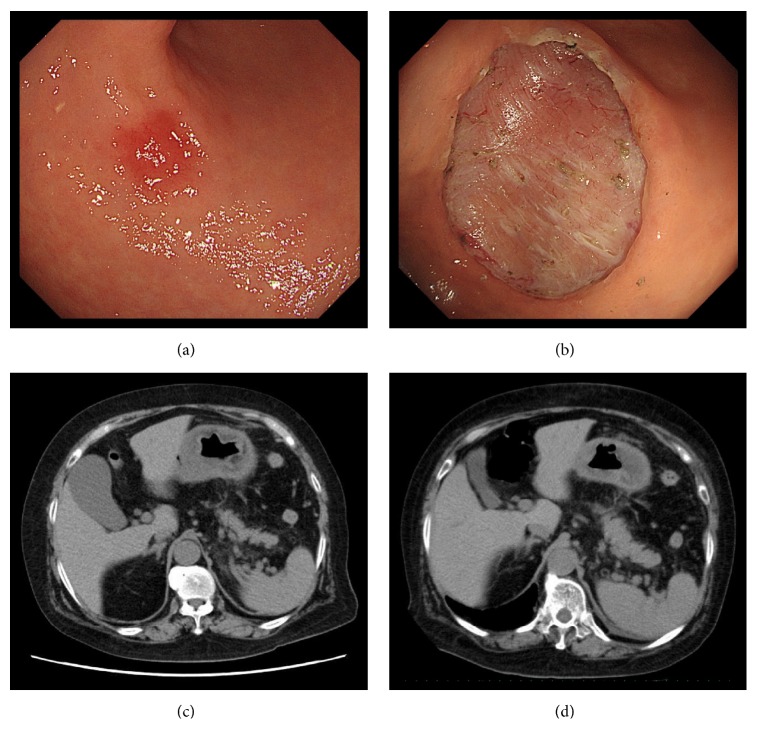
(a, b) ESD. (c) Abdominal CT (day 3 post-ESD). (d) Abdominal CT (day 20 post-ESD).
